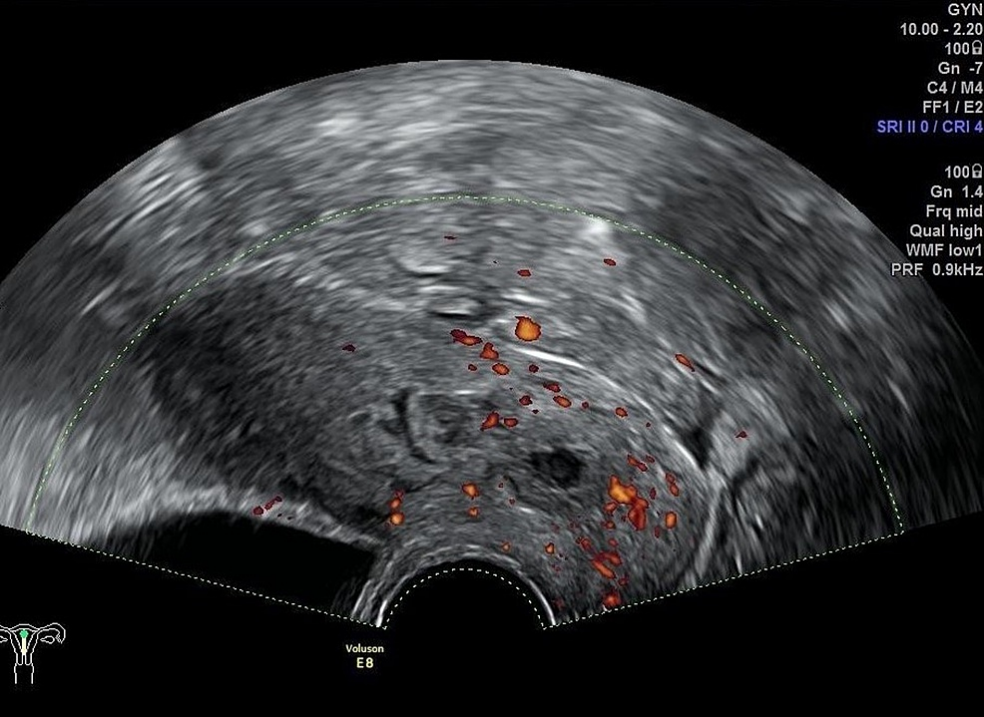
Transvaginal ultrasound (sagittal plane) – "8" or hourglass uterine shape caused by cervical enlargement
